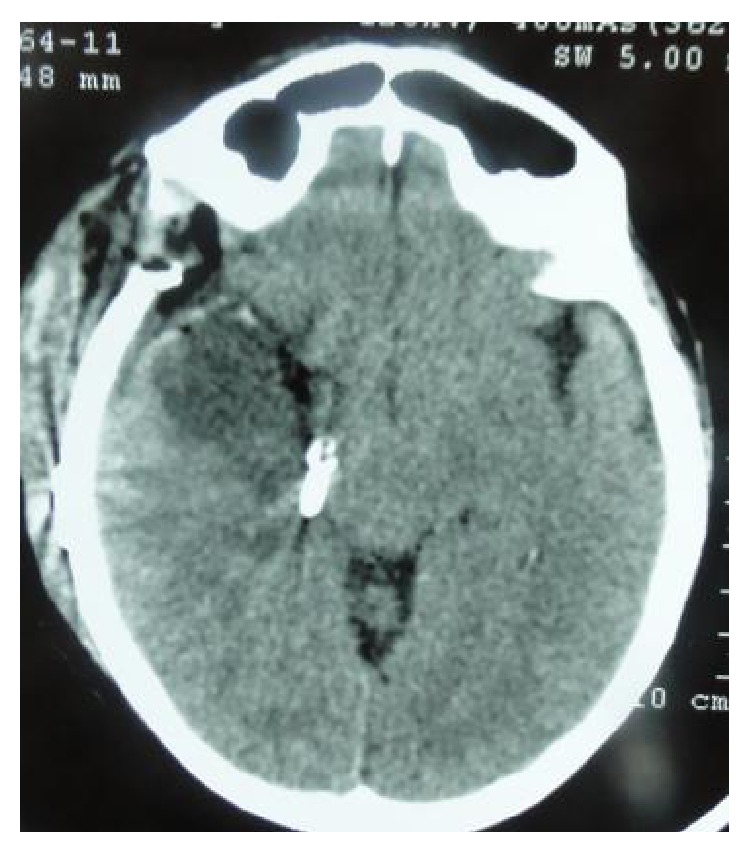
A postoperative CT scan.
